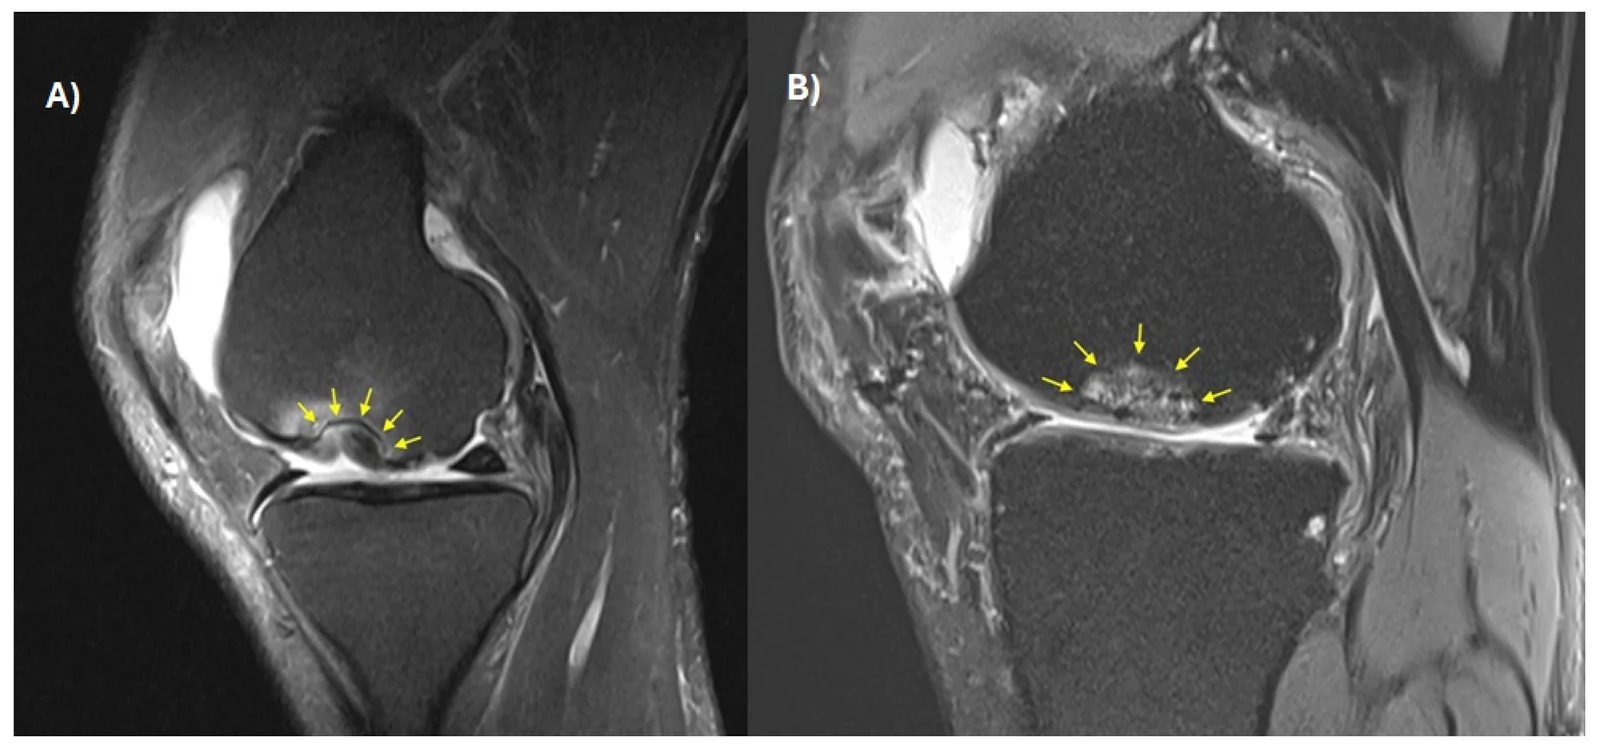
(A) The spot of osteonecrosis of the medial femoral condyle (MFC) visualized in MRI using PD Fat Sat sequence. (B) The spot of regenerated osteochondral tissue at MFC visualized in MRI using PD Fat Sat sequence 7 years after implantation autologous morselized bone chips and a collagen scaffold soaked with WJ-MSCs in dry arthroscopy technique.
